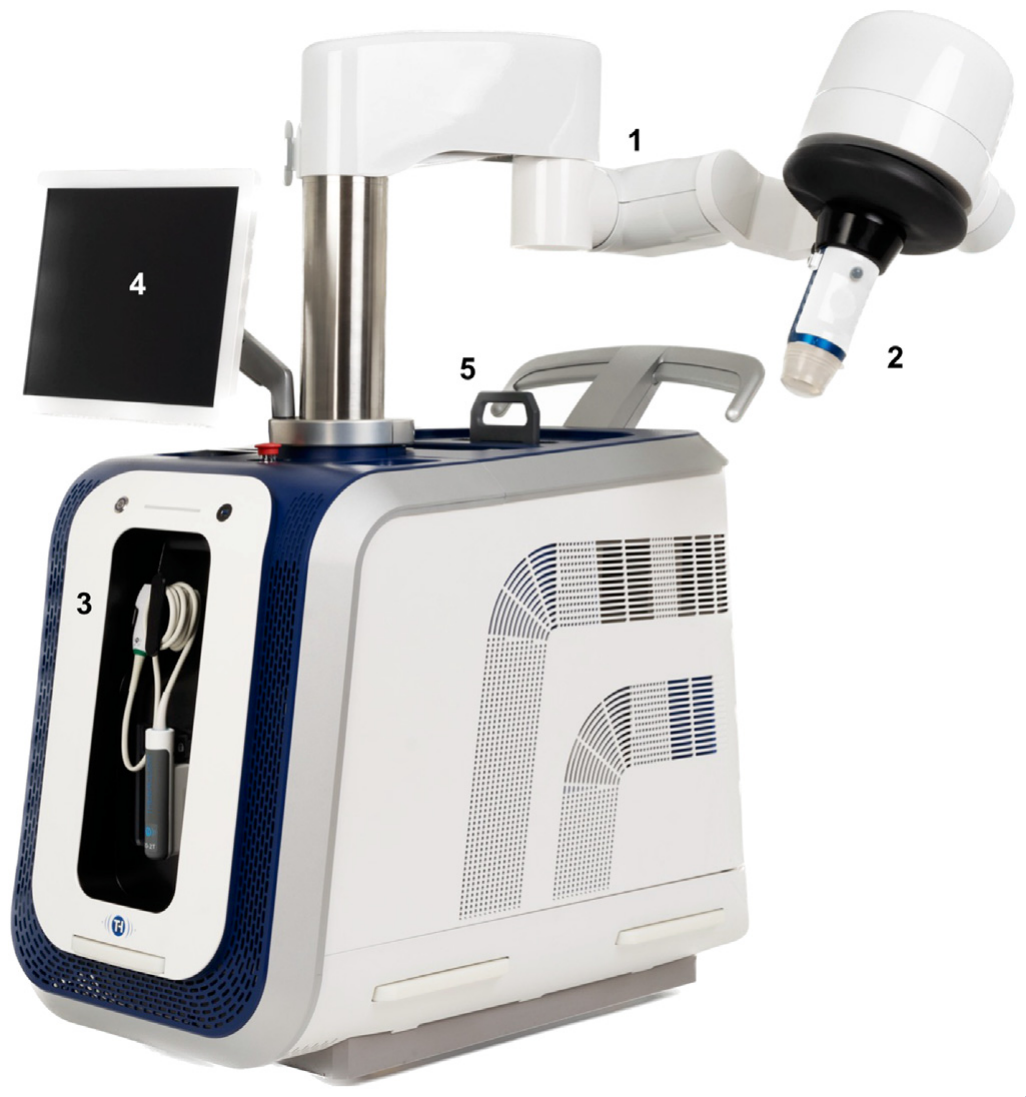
SONOVEIN HD version device. (1) Robotic arm. (2) Visualization and treatment unit (VTU). (3) Ten-MHz linear array ultrasound (US) handheld probe. (4) Touch screen control console. (5) Cooling system (courtesy of Theraclion).
